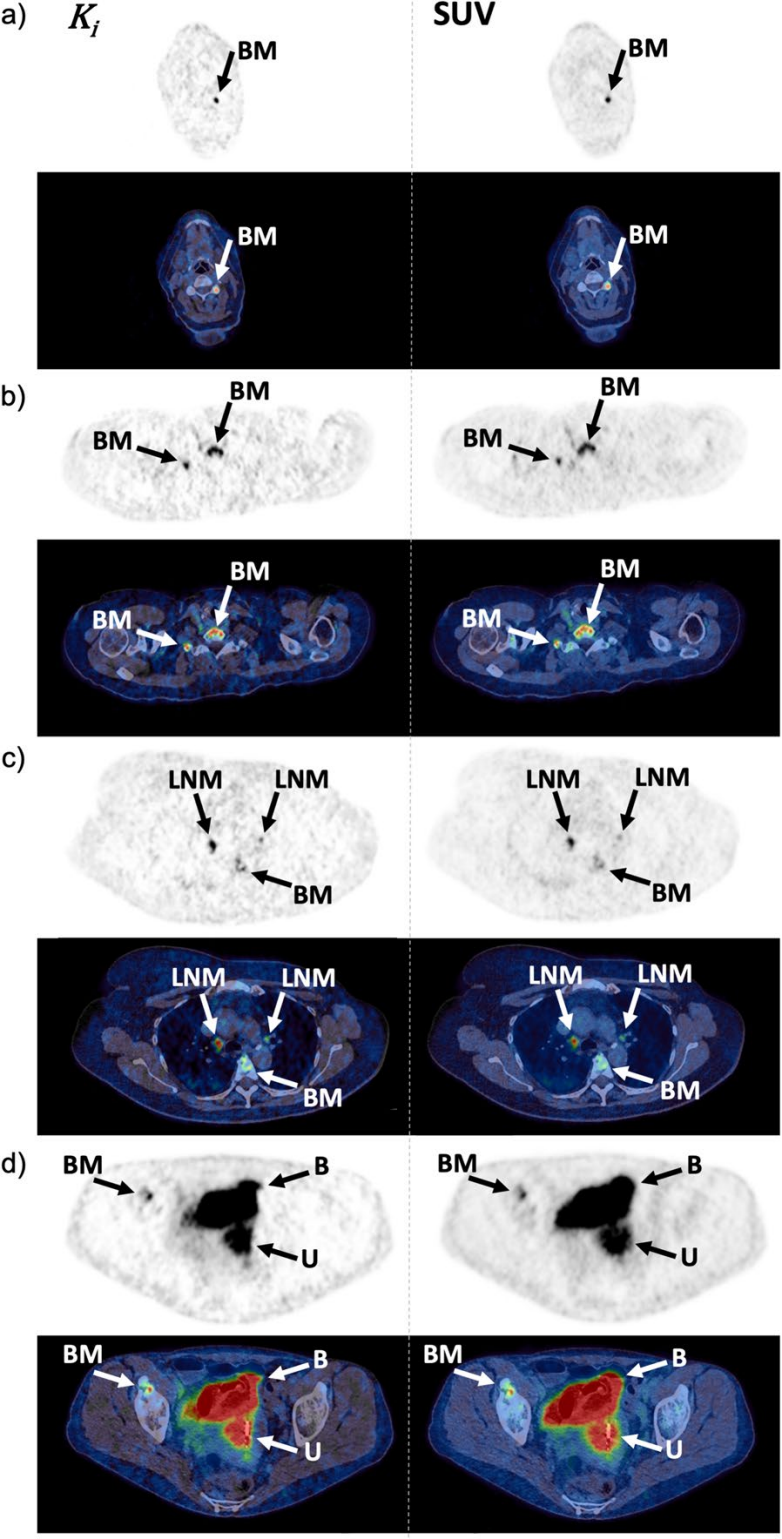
Examples of the better TBR and CNR for bone metastases (BM) and LN metastases (LNM) in $${K}_{i}$$ images compared to SUV images. Metastases were located in a C5, b C7, c T5 and mediastinal lymph nodes, and d pelvic bone, where the bladder (B) and uterus (U) were also present
